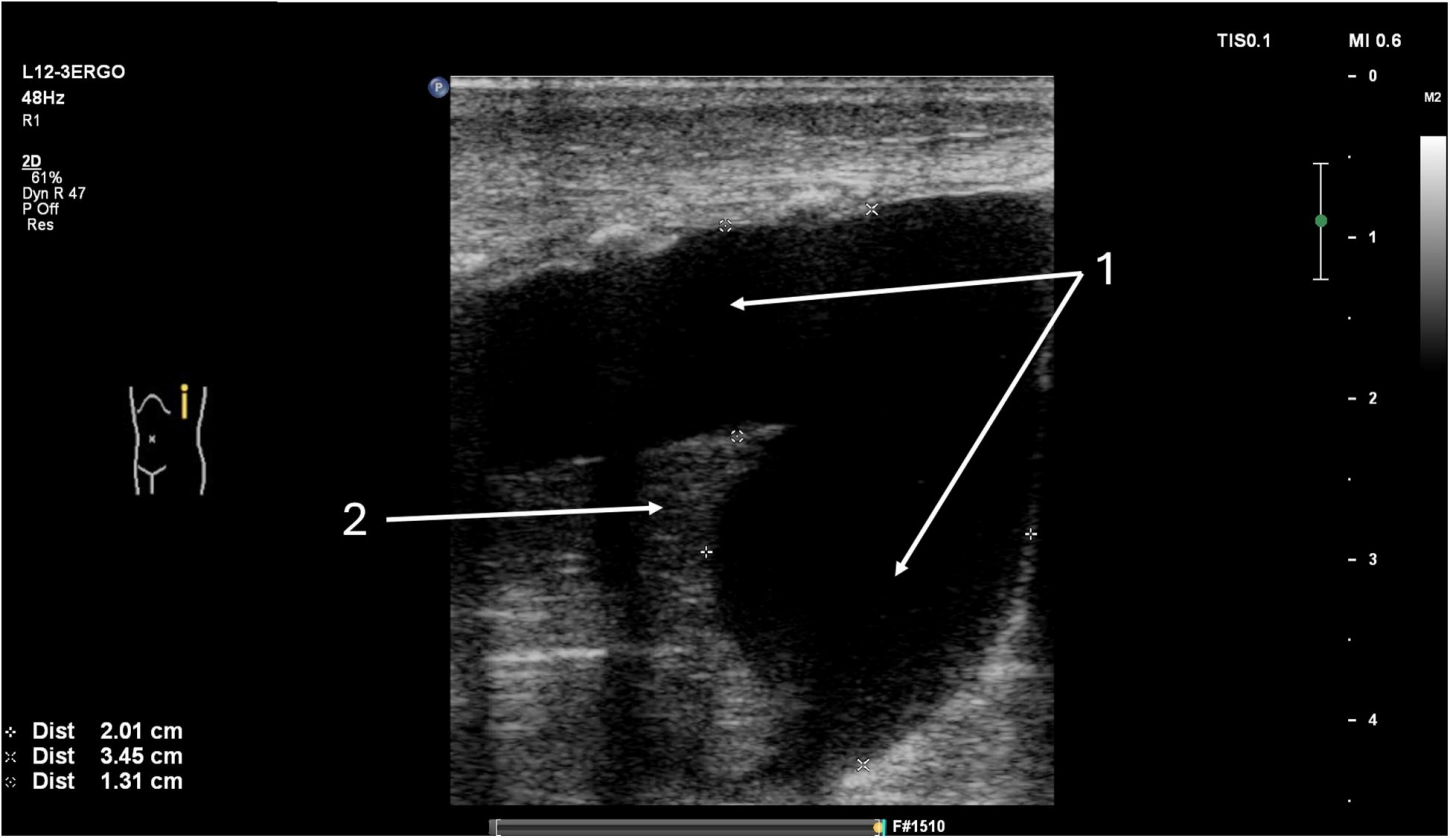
Fluid detected in the left pleural cavity, longitudinal lung ultrasound. The patient is a 10-day-old newborn with coarctation of the aorta following cardiac surgery involving repair of the aortic arch. (1) Large volume of fluid present in the left pleural cavity. (2) Complete atelectasis of the lower lobe of the left lung.
